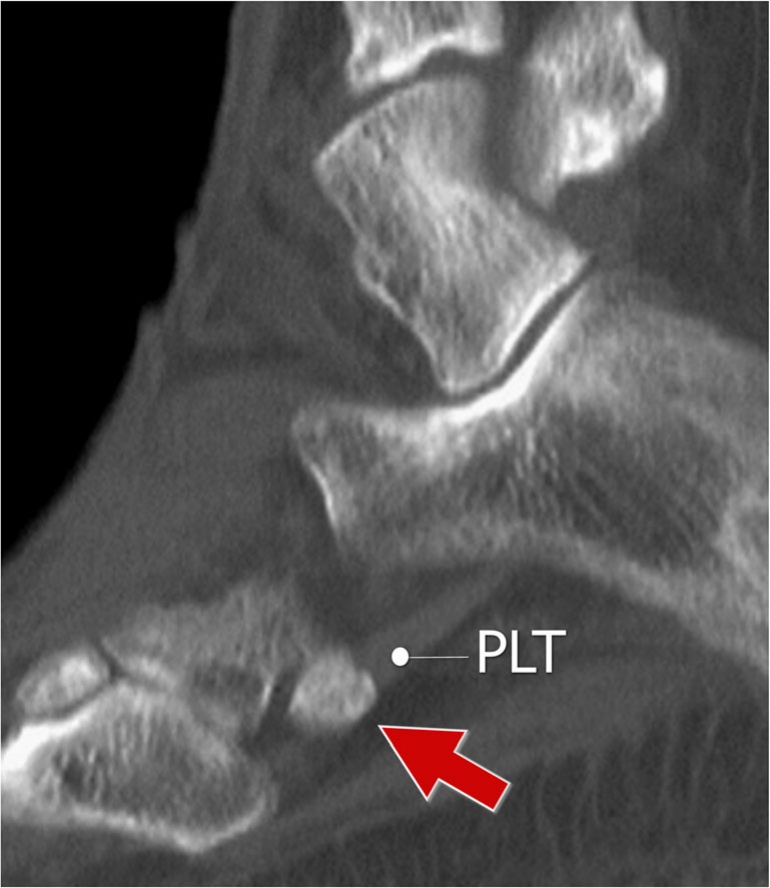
Normal os peroneum – computed tomography. Sagittal reconstruction along the PLT allows a precise evaluation of the OP (*arrow*)
